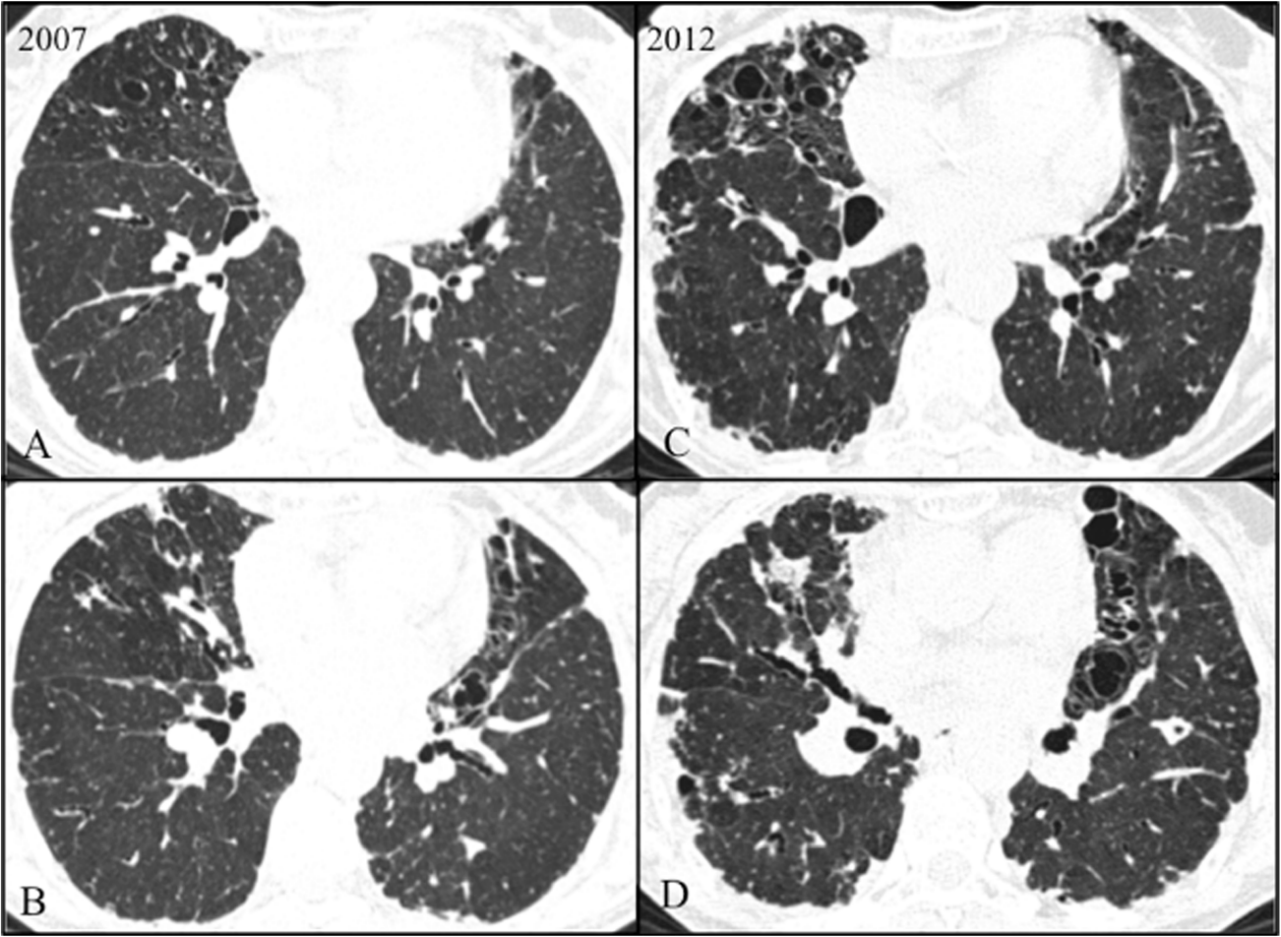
Axial HRCT scans in 2007 (a–b) and 2012 (c–d) in patient 2 show multiple cystic bronchiectasis in the middle right lobe and lingula with worsening between 2007 and 2012
